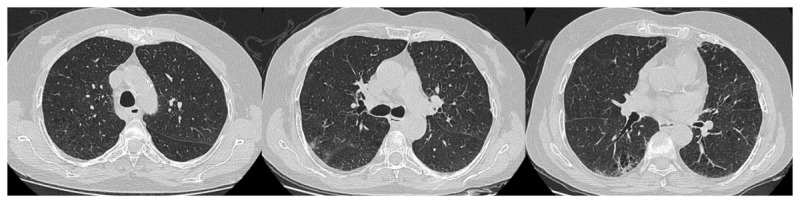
RT-PCR –, CO-RADS 4: This 70-year-old female patient had chronic obstructive pulmonary disease. He presented to the emergency department with a respiratory distress. There are no findings compatible with COVID-19 except d-dimer: 1060 ng/mL CRP: 126 mg/L in laboratory parameters. He was admitted to the intensive care unit with the diagnosis of COVID-19, with negative SARS-CoV-2 PCR test and unilateral ground glass oppacities with emphysema and bronchiectasis on chest CT. It was evaluated as CO-RADS 4. PCR tests on day 1 and day 6 are negative. He died on the 9th day of his hospitalization.
